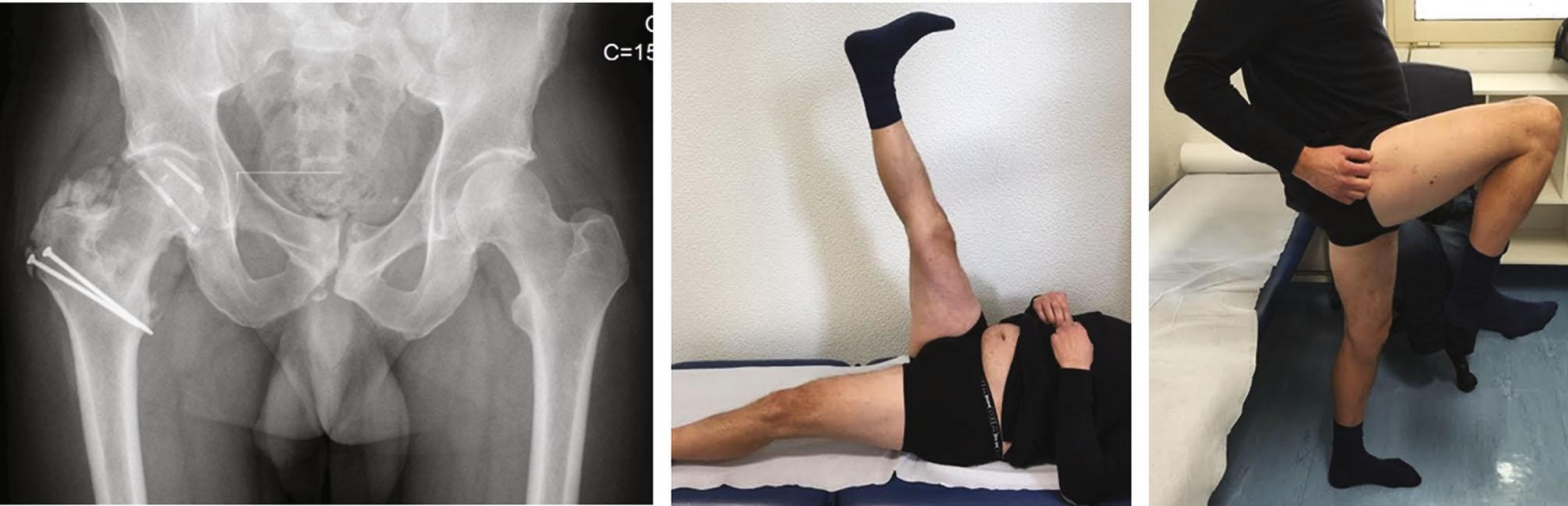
Simple anteroposterior radiograph showing supratrochanteric heterotopic ossification at 3 years of follow-up, with a good active motion of the hip and painless hip flexion.
